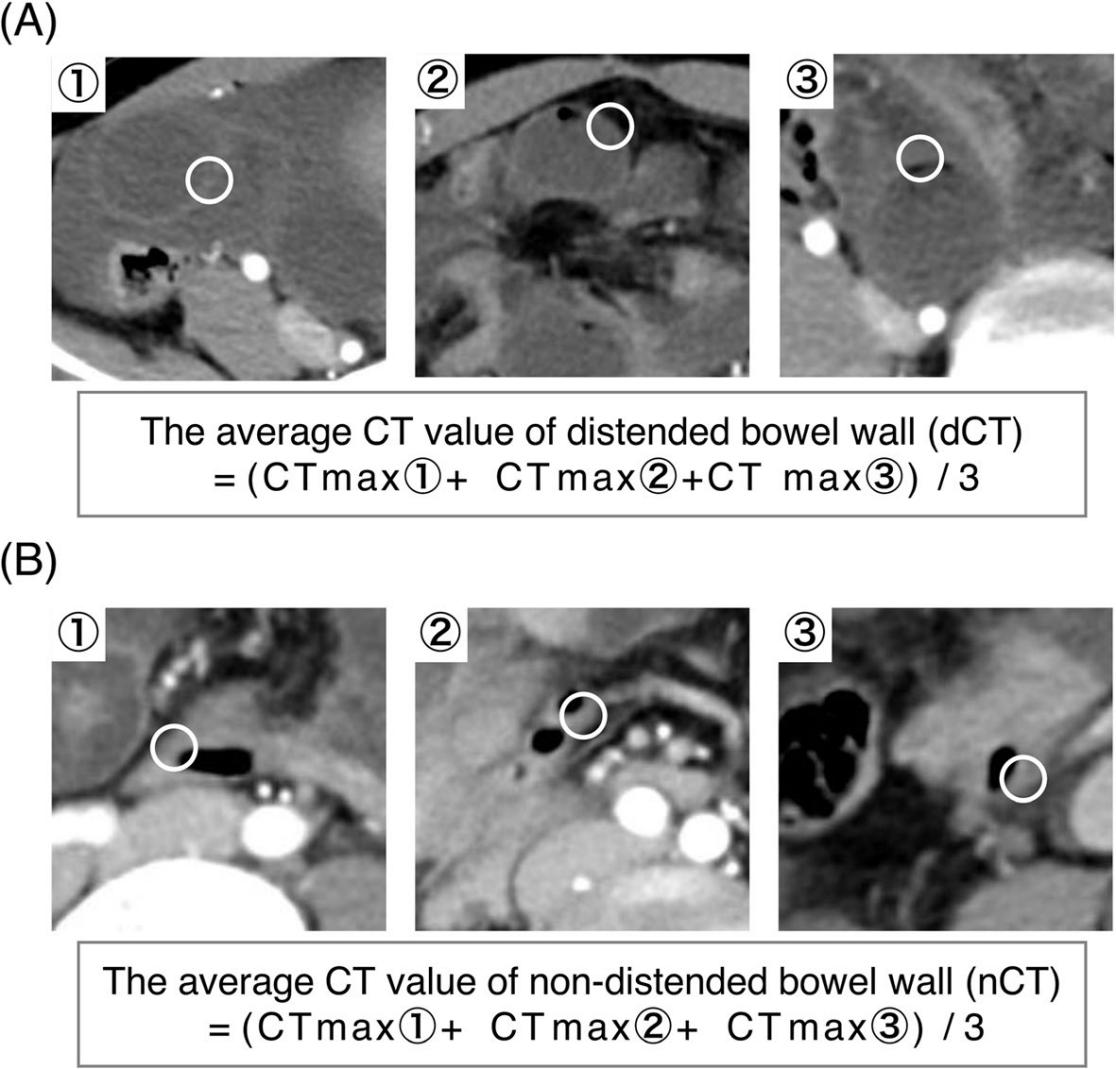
Measurement method of the CT value of the small bowel wall. 10 mm-white circles were shown as region of interest (ROI). The small-bowel wall was contained in ROI. The highest CT value of ROI was set as the CT value of the wall. Three different sites were measured. A Representative figure of the measurement of distended small bowel. The average value was calculated as the average CT value of distended bowel wall (dCT). B Representative figure of the measurement of non-distended small bowel. The average value was calculated as the average CT value of non-distended bowel wall (nCT). CT, computed tomography. ROI, region of interest
